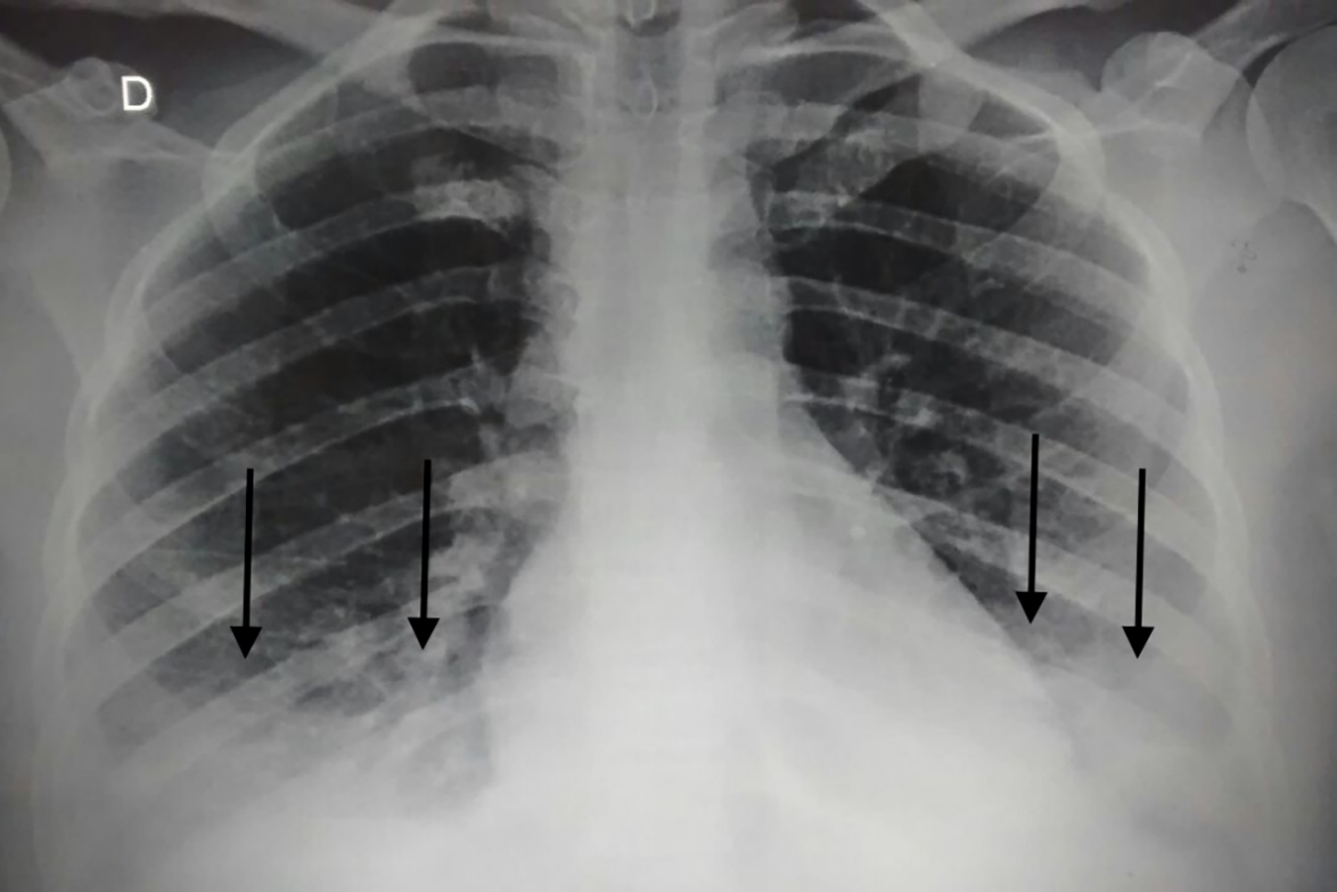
Antero-posterior chest X-ray of the patient during hospitalization, revealing bilateral basilar consolidation, arrows.
